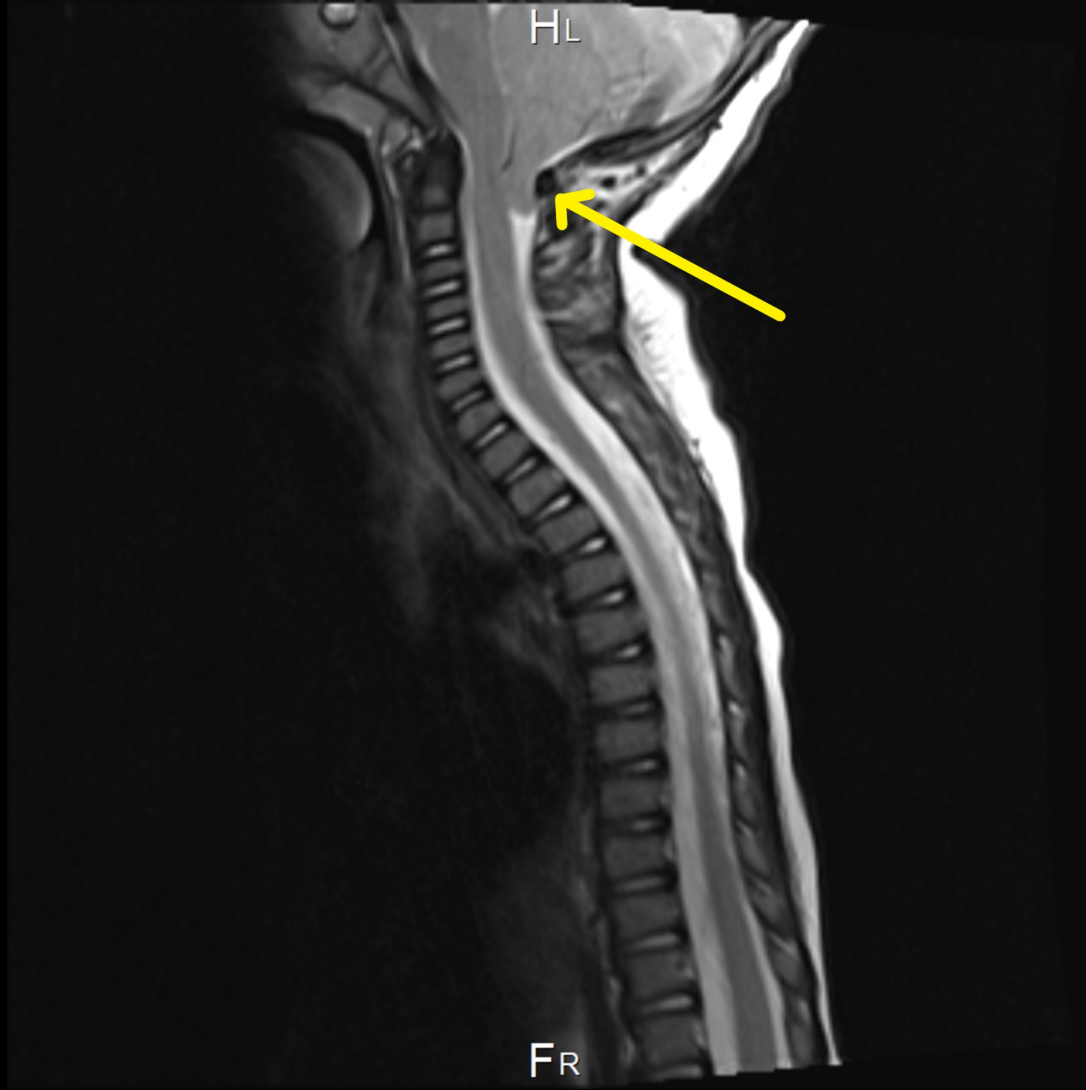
Whole spine MRI showing a significant descent of the cerebellar tonsils through the foreman magnum to the left of C2
